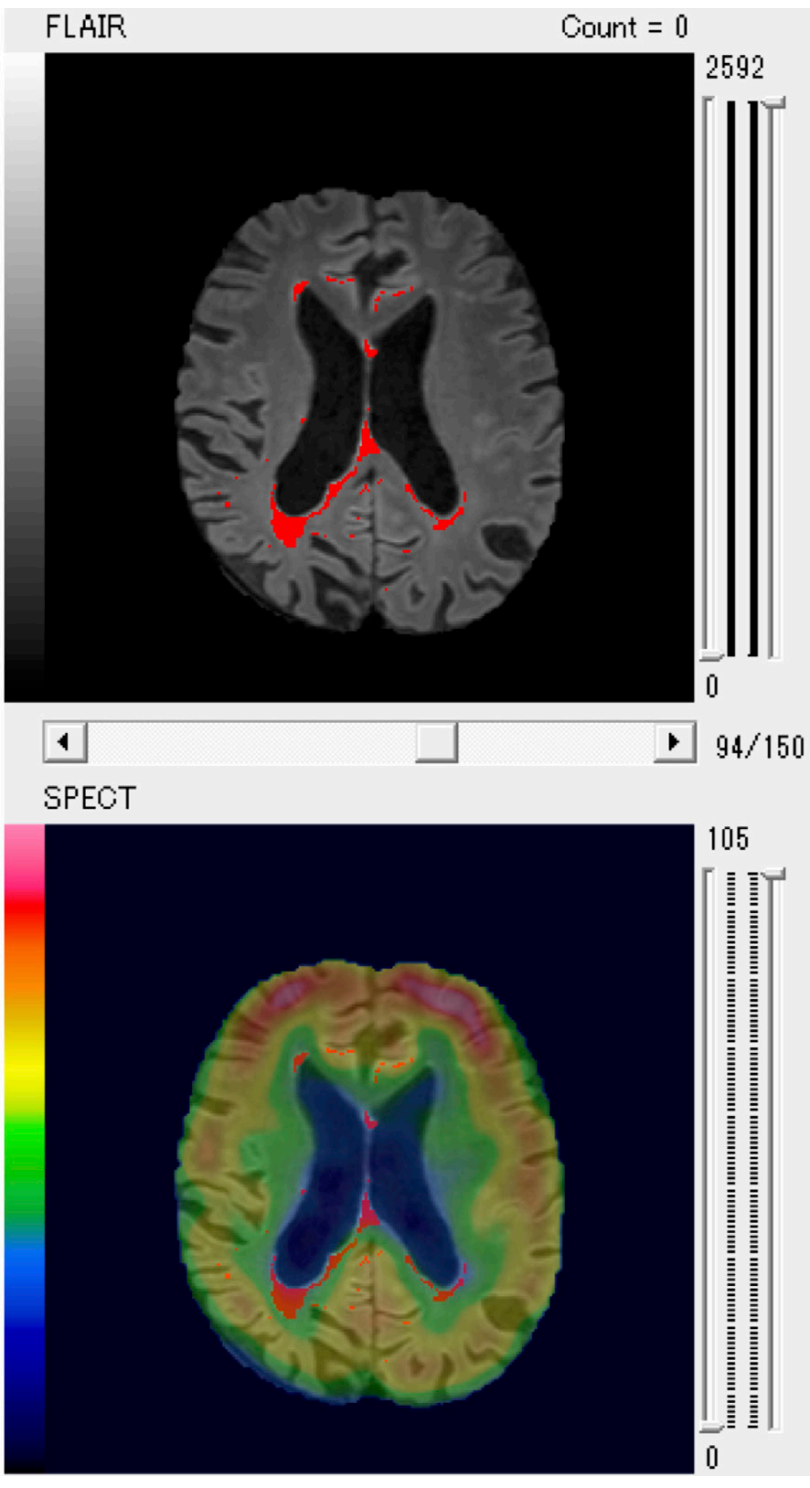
A patient with larger posterior white matter hyperintensity (WMH) volume and decreased regional cerebral blood flow of parietal region shown as a typical example of fused WMH and single-photon emission computed tomography (SPECT) images for quantitative volume analysis.
